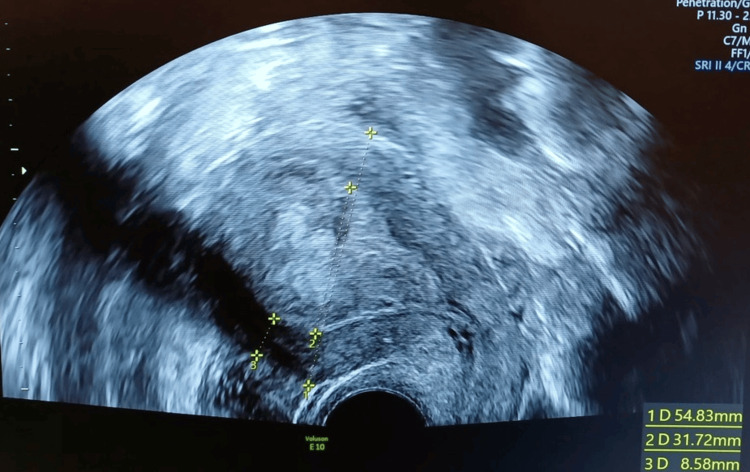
Transvaginal ultrasound Line 2: endometrial thickening
